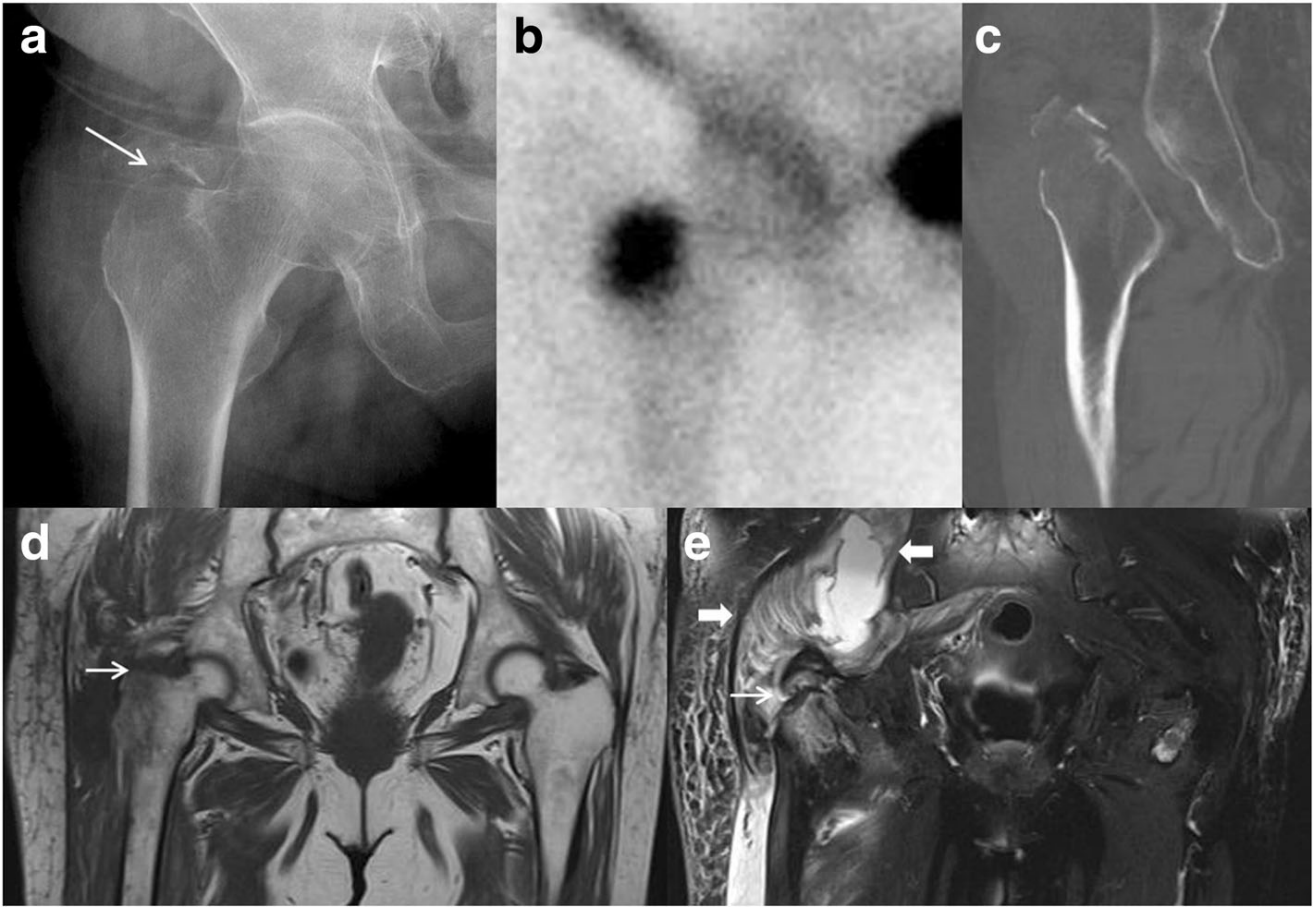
Case of a 72-year-old who presented at the outpatient department with right hip pain after slipping at home. a A plain radiograph of the right hip shows only an isolated greater trochanter fracture (*arrow*). b A bone scan obtained 3 days after the trauma shows radionuclide predominantly concentrated in the greater trochanter. c A computed tomography scan shows no occult intertrochanteric fracture. d A T1-weighted coronal image shows a linear low-signal intensity focus (*arrow*) indicating a fracture. e A T2-weighted coronal image shows a fracture surrounded by high signal intensity (*arrow*) and abnormal signal in the muscles around the hip. These findings are compatible with interstitial hemorrhage and hematoma (*bold arrows*)
